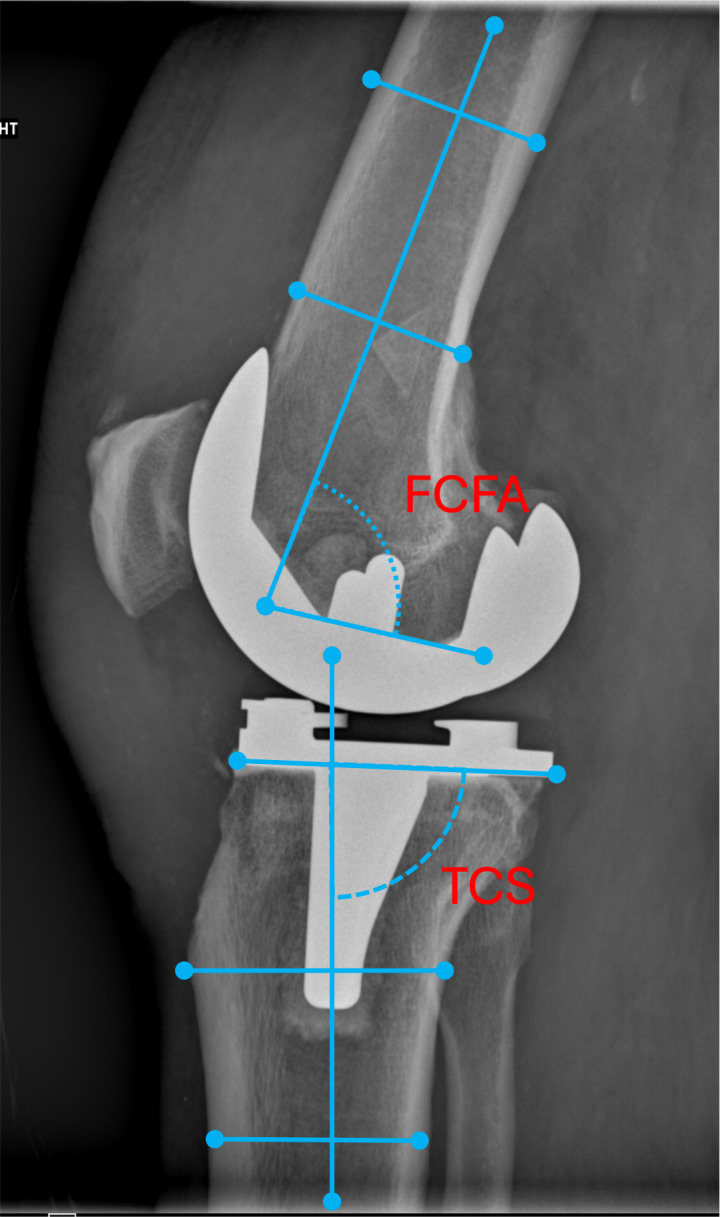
Radiographic measurements of TKA component placement in the sagittal plane. In the sagittal plane, the femoral component is assessed by femoral component flexion angle (FCF), defined as the angle between the distal femoral axis in the sagittal plane and a line perpendicular to the distal surface of the femoral component. A femoral component flexion angle of 0–8° is usually aimed. Tibial component placement is assessed by tibial component slope (TCS), defined as the angle between the line that is perpendicular to the bottom of the tibial plate and the tibial shaft axis in the sagittal plane. TCS measurements must consider that some TKA types have the posterior slope built into the implant design.
